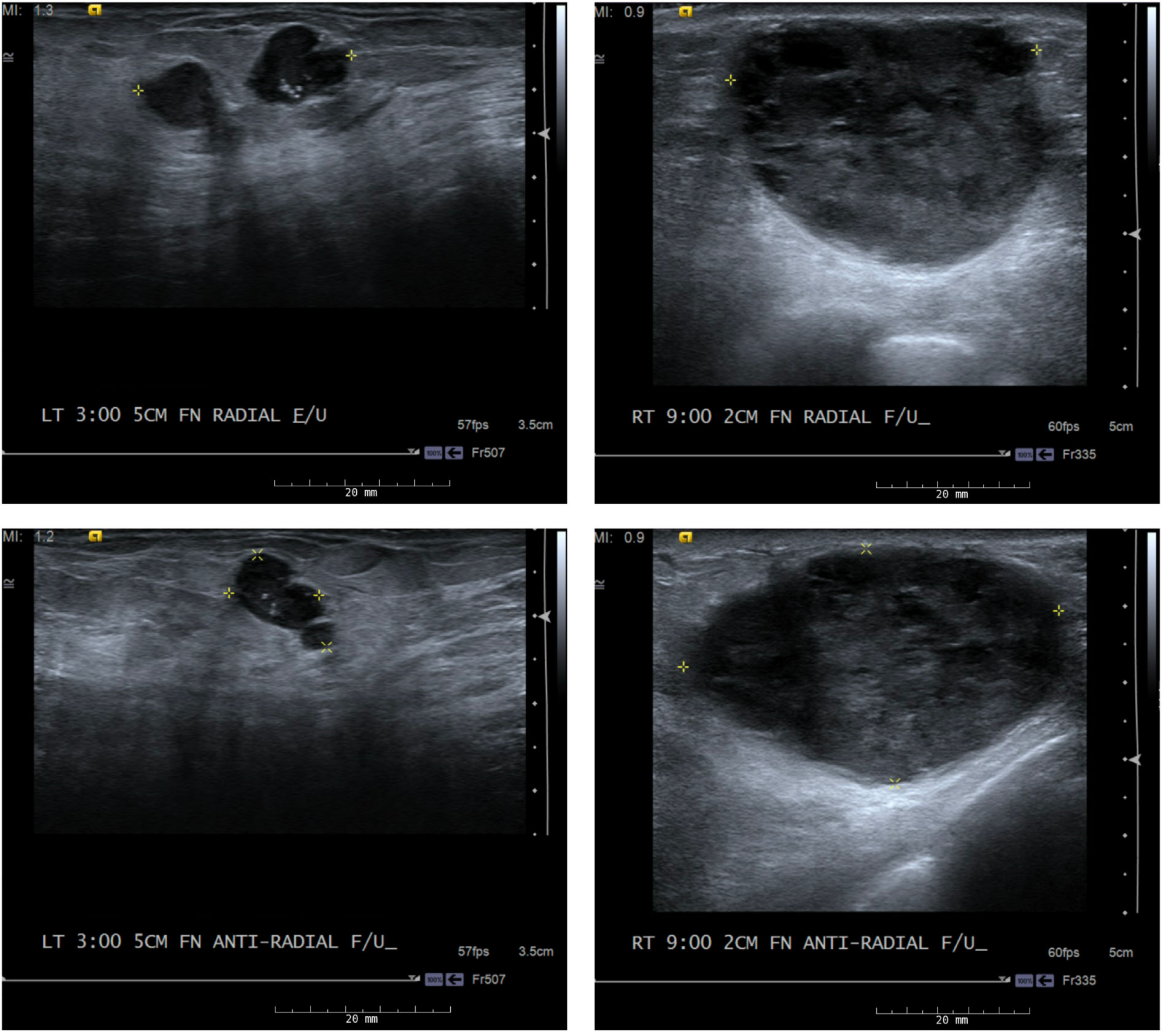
Follow‐up bilateral breast ultrasound images, 11 weeks from initial diagnostic mammogram. Top left: left breast, radial. Top right: right breast, radial. Bottom left: left breast, antiradial. Bottom right: right breast, antiradial
